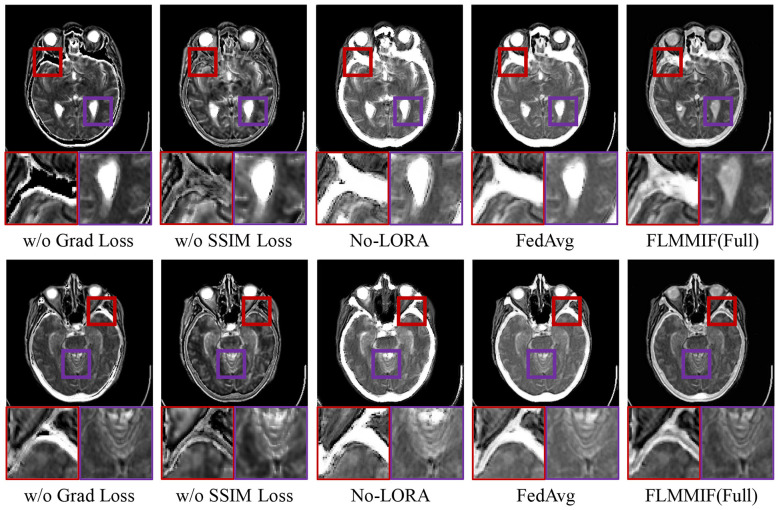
Quantitative assessment of ablation study on CT-MRI dataset.
